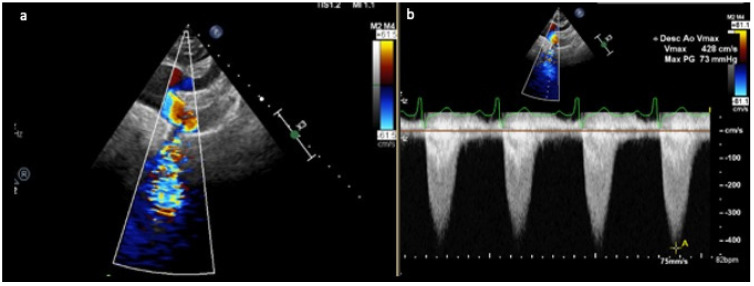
(a) Suprasternal view demonstrating a focal area of narrowing of the thoracic aorta distal to the origin of the left subclavian artery with associated flow turbulence on colour flow Doppler. (b) Continuous wave Doppler shows increased velocity across the site of coarctation.
